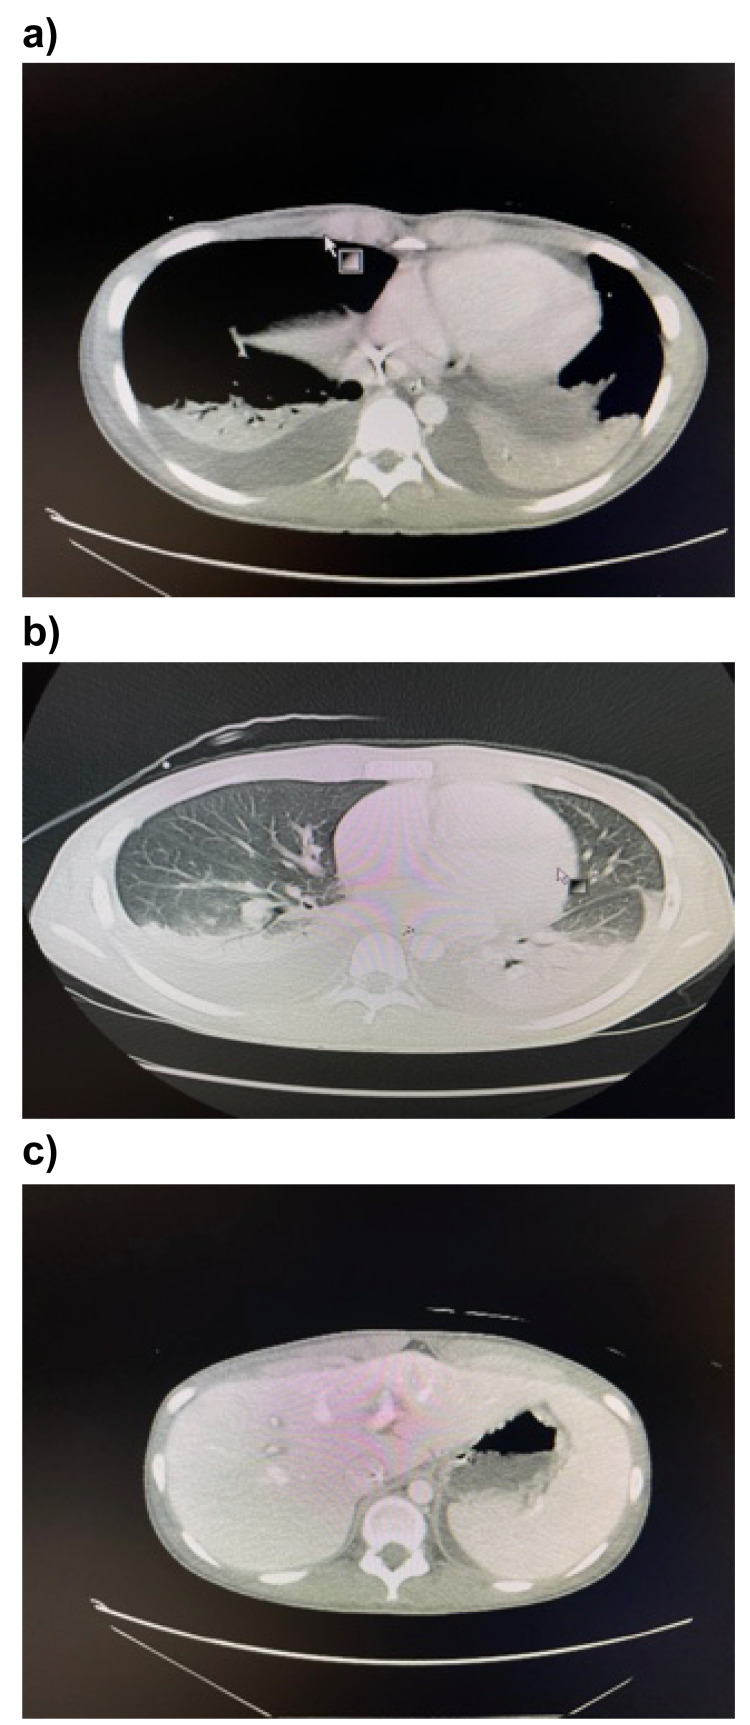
Contrast-enhanced thoracoabdominal computed tomography findings on admission. a) Bilateral pleural effusions. b) Consolidation in both the lungs. c) Splenomegaly.
